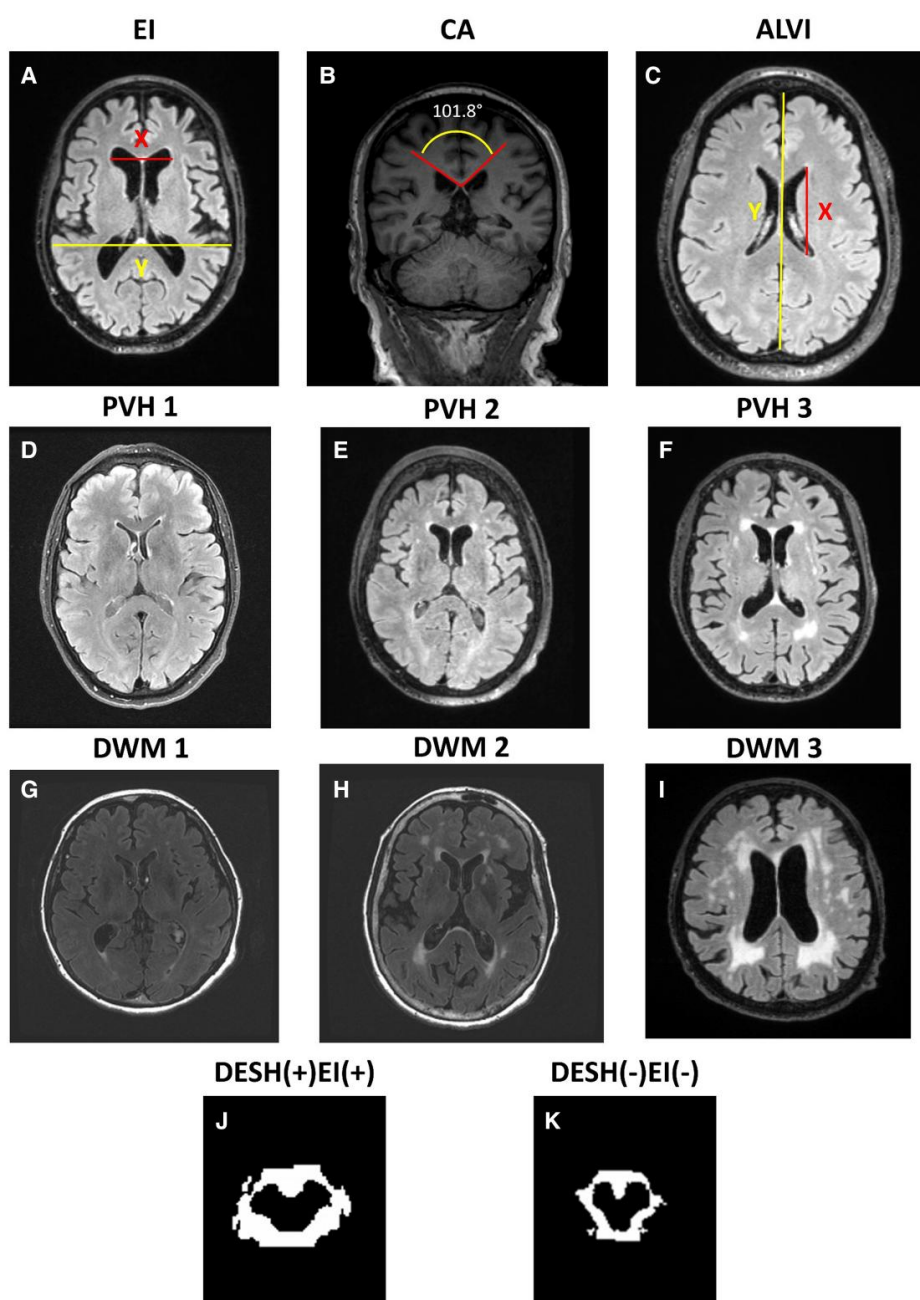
Examples of manually MRI measurements. (A) Evans’ index (EI): maximum width of the frontal horns of the lateral ventricles (X)/the maximal internal diameter of the skull at the same level (Y) = 0.30. (B) Callosal angle (CA): measured on a coronal image perpendicular to the anterior commissure–posterior commissure plane at the level of the posterior commissure. (C) Anteroposterior diameter of the lateral ventricle index (ALVI): anteroposterior diameter of lateral ventricle (X)/maximal width of the anteroposterior inner diameter of the skull (Y) = 0.33. (D–F) Fazekas scale of PVH 1–3: 1 = ‘caps or pencil-thin-lining’, 2 = ‘smooth halo or thin band’, and 3 = ‘irregular PVH extending into the deep white matter or broad band’. (G–I) Fazekas scale of DWM, grade 0 = ‘absent’, 1 = ‘punctate’, 2 = ‘beginning of confluence’, and 3 = ‘confluent’. (J, K) Example imaging of manually measured cisterns in D+E+ and D−E− participants. Cistern areas include crural, ambient and quadrigeminal cisterns.
